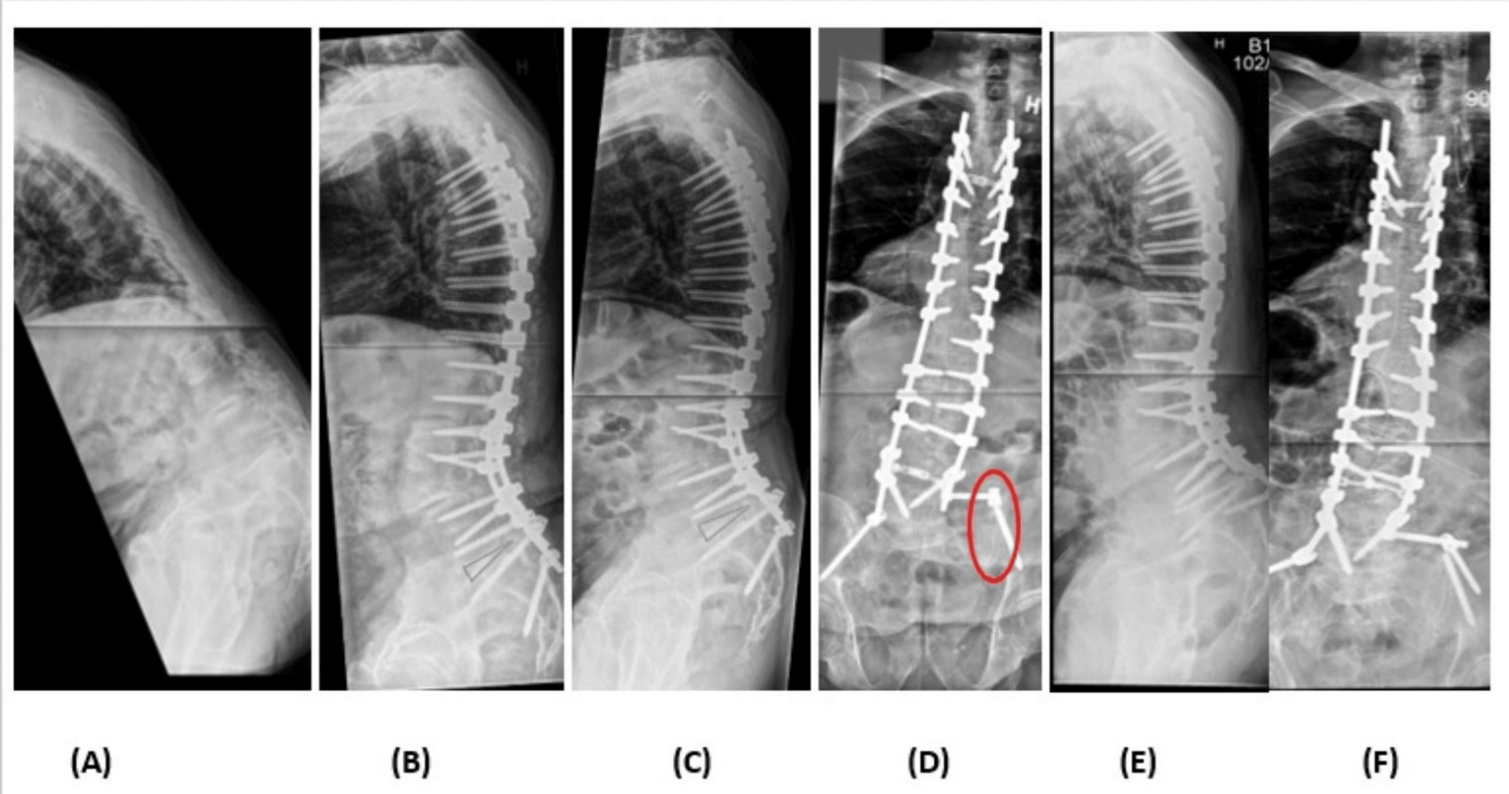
Case 1 A 71-year-old male was referred due to severe sagittal imbalance and back pain (A). He had previously undergone multiple decompression surgeries due to degenerative lumbar disease. At the time of referral, the patient presented with bilateral crista-costae collision, localized kyphosis in the lumbar region, and SVA of 285 mm. The patient underwent posterior instrumentation from Th4 to the pelvis, PSO at L3, and supplemental ALIF at L5/S1 (marked in blue), with an improvement of the sagittal imbalance (B). After 315 days, the patient was evaluated in the outpatient clinic due to acute back pain. CT scan revealed a broken iliac screw (red circle) and pseudarthrosis at the PSO level (C and D). The patient underwent revision surgery with replacement of both iliac screws, revision of pseudarthrosis at the PSO level, and additional SPO L1/L2 (E and F). SVA: sagittal vertical axis, PSO: pedicle subtraction osteotomy, ALIF: anterior lumbar interbody fusion, CT: computed tomography
